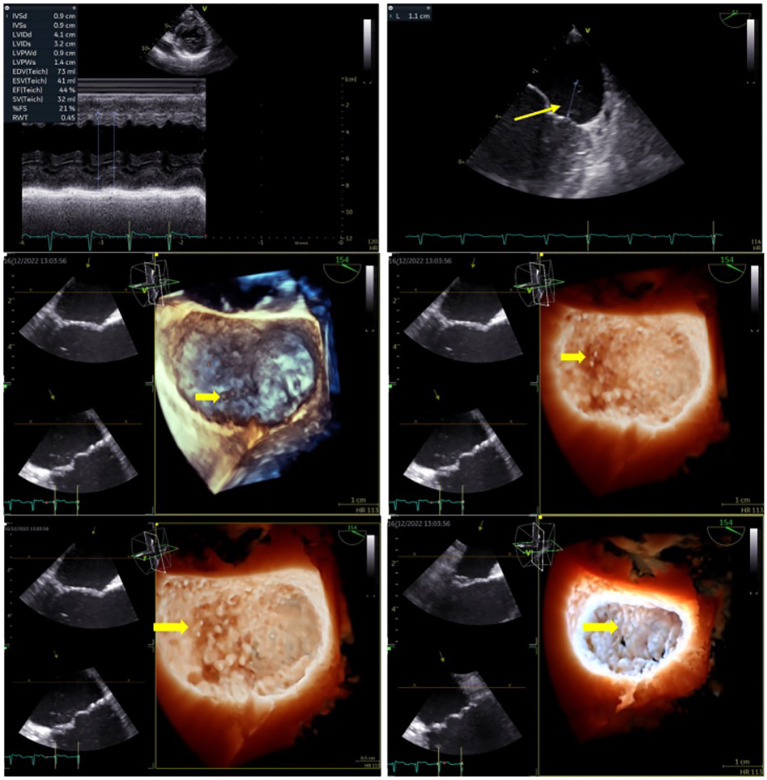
Transesophageal echocardiography findings consistent with Loeffler endocarditis.
